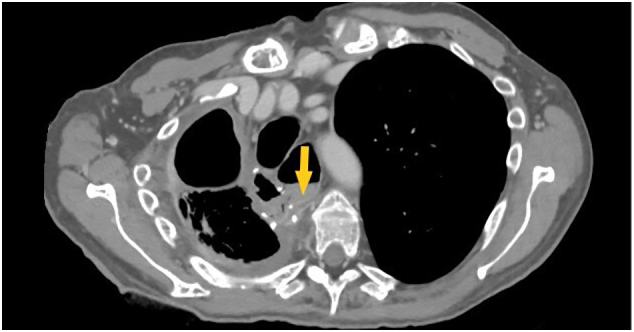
Postoperative CT scan showing leak of CE.
